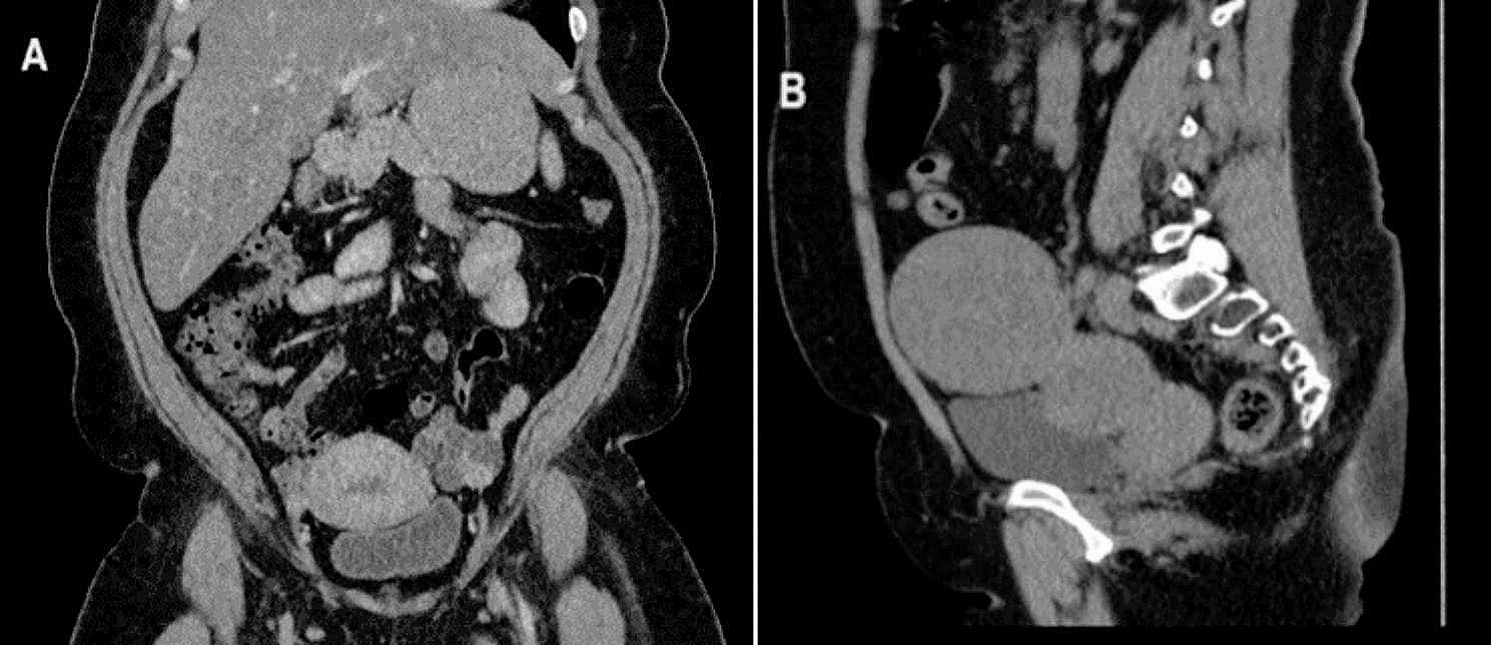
CT abdomen pelvis. (A) 3.8 × 2.7 × 4 cm complex left adnexal mass containing thick septations and enhancing soft tissue component. (B) 8.8 × 9 × 9.5 cm well defined heterogenous soft tissue mass in relation to left adnexa
